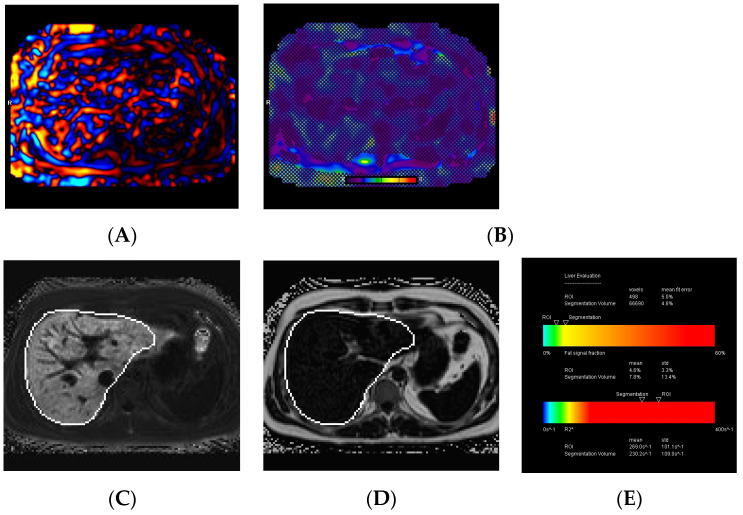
A 23-year-old female with juvenile hereditary hemochromatosis. The images show poor-quality disorganized mechanical waves (A), and a non-diagnostic elastogram (B), on MRE due to signal degradation from the iron overload. MRI-PDFF map (C), R2* map (D), and the liver evaluation table (E), demonstrate mild steatosis (7.8%) and moderate iron overload (R2* of 230.2 s^−1^ and a LIC of 7.4 mg/g) based on segmentation.
